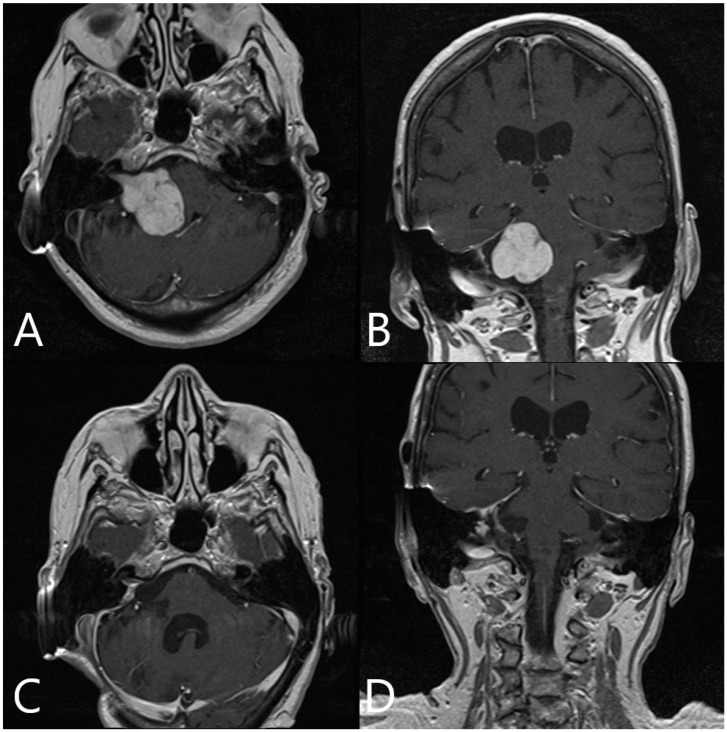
Patient No. 41. Preoperative (A) axial and (B) coronar T1-weighted post-contrast MRI with postoperative (C) axial and (D) coronar T1-weighted post-contrast MRI, depicting subtotal resection of the tumor and slight contrast enhancement along the course of the facial nerve.
